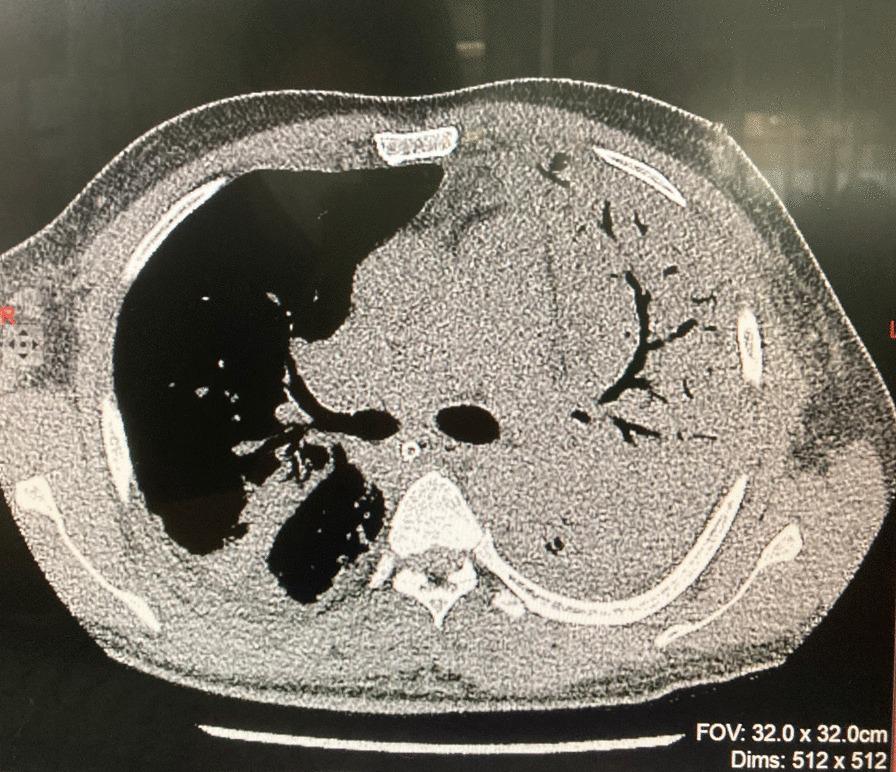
High resolution computed tomography chest showing dense consolidation of left lung
